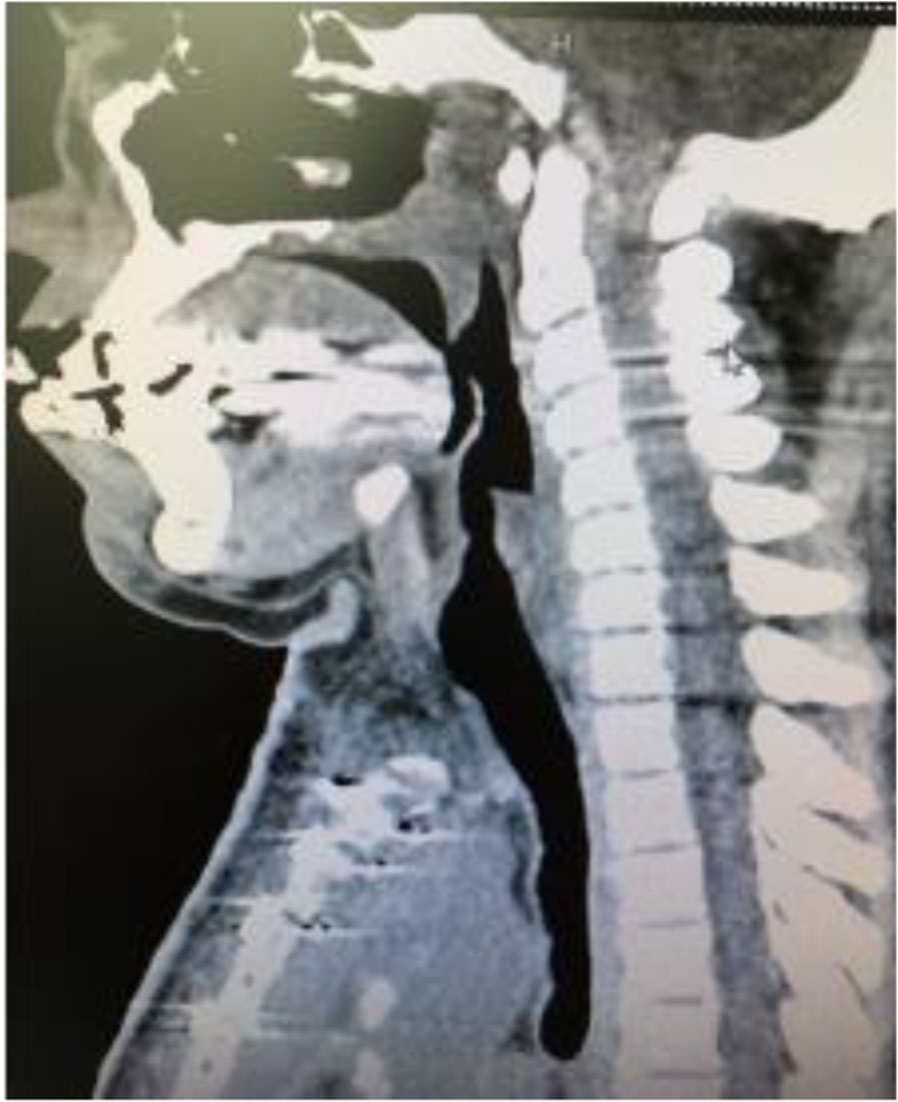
Cervical CT revealed that cervical vertebrae comprised a straight neck, glottis were present at the level of the sixth cervical vertebra, and the airway patency was maintained
